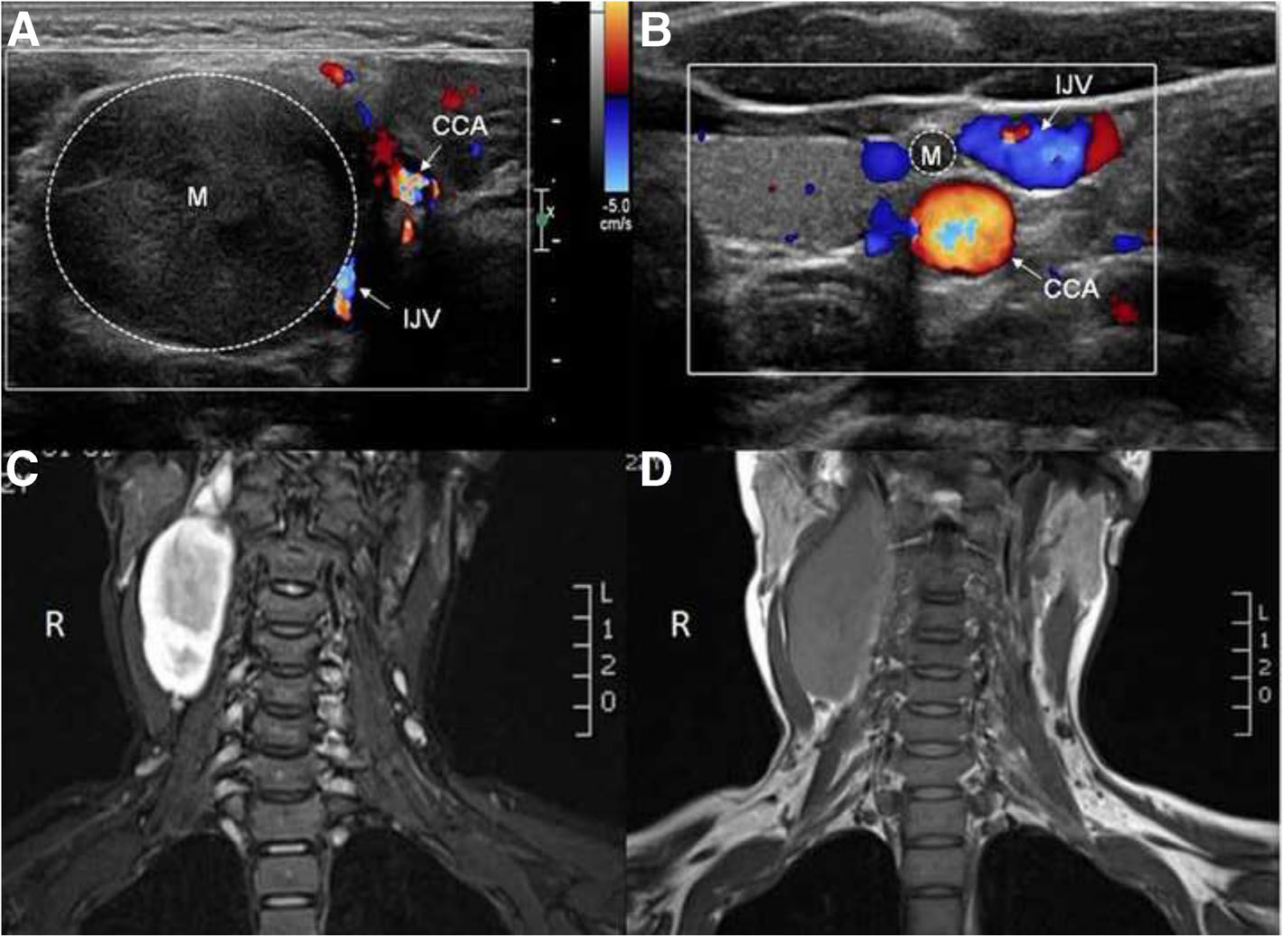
a Axial sonogram (5–12-MHz) showing a hypoechoic mass (M, dash line) centered on the right carotid sheath, between the internal jugular vein (IJA) and the common carotid artery (CCA). b Axial sonogram (5–12-MHz) showing a hypoechoic mass (M, dash line), vagus nerve (arrow) and common carotid artery (CCA). c The coronal T2-weighted MR imaging shows a 7.7 cm × 4.1 cm × 3.0 cm well-defined hyperintense mass adjacent to the right internal carotid artery. d The right tumor appeared hypointense on coronal T1-weighted MR imaging
